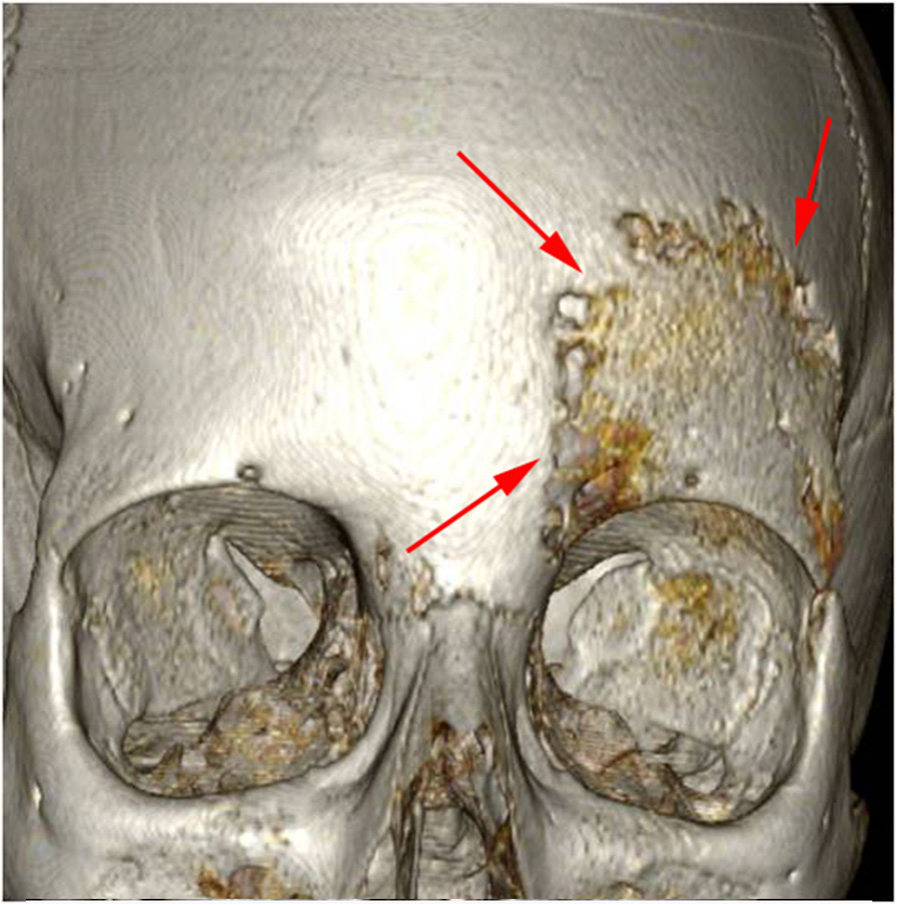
CT scan three-dimensional reconstruction
